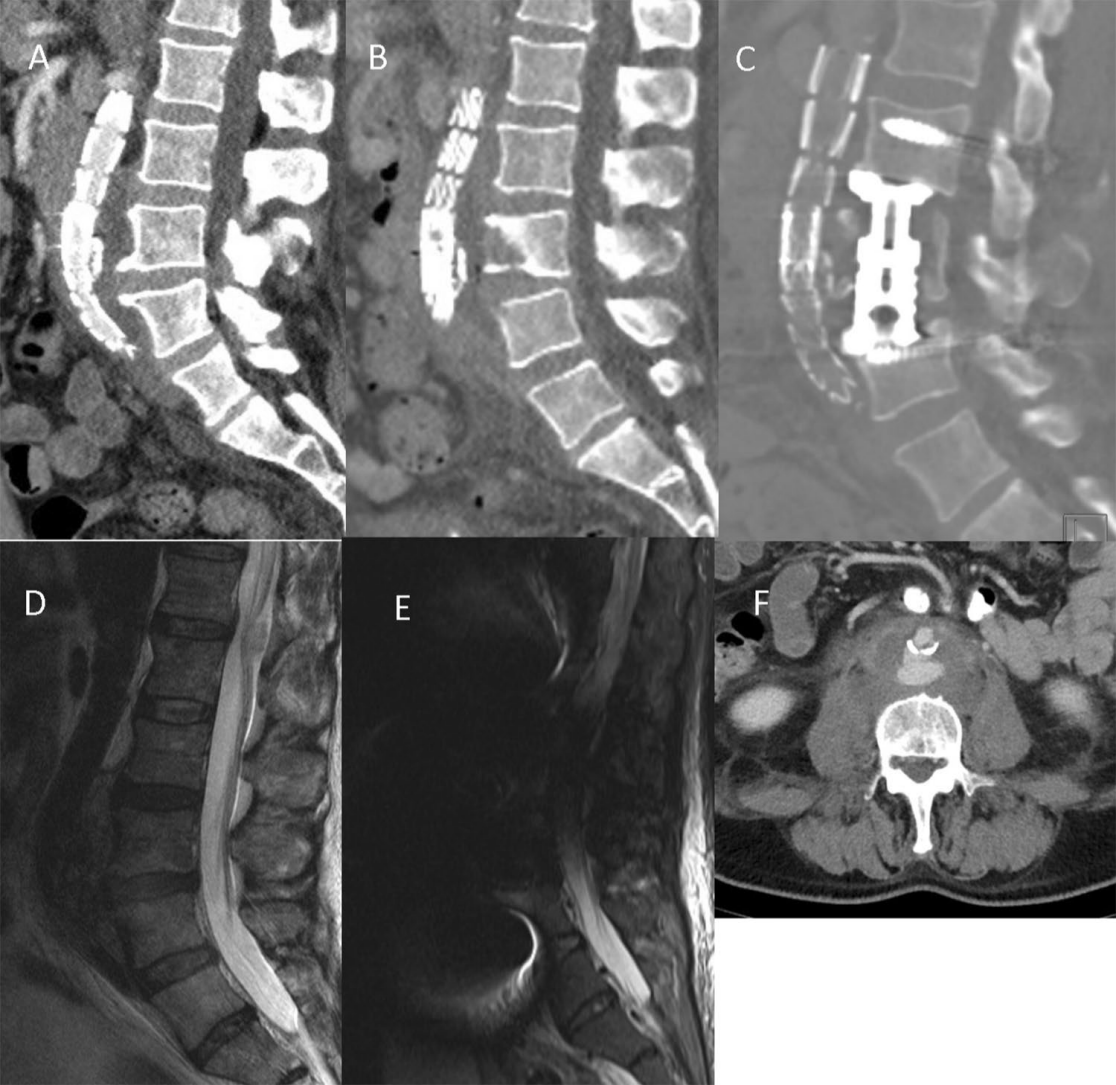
Computed tomography (CT) scan at a the beginning under antibiotic therapy and progressive anterior bony destruction and b after posterior instrumentation and anterior vertebral body replacement c in sagittal plane. Magnetic resonance imaging (MRI) at d the beginning of clinical symptoms and e after spinal surgery. f Axial CT scan with perifocal abscess around endovascular aortic repair (EVAR)
